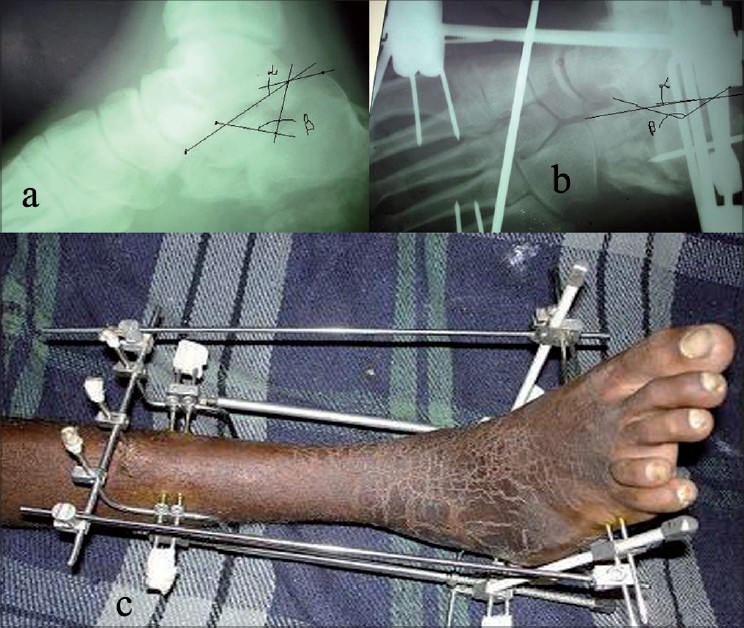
(a) Preoperative X-ray of the ankle (Lateral view) showing the type of fracture pattern and extent of comminution with significantly altered α and β angles of calcaneum. (b) Immediate postoperative X-ray of the ankle (Lateral view)showing fixator in position with on table partially corrected angles of calcaneum (c) Clinical photograph of the same shows the JESS fixator in position without any skin incision
